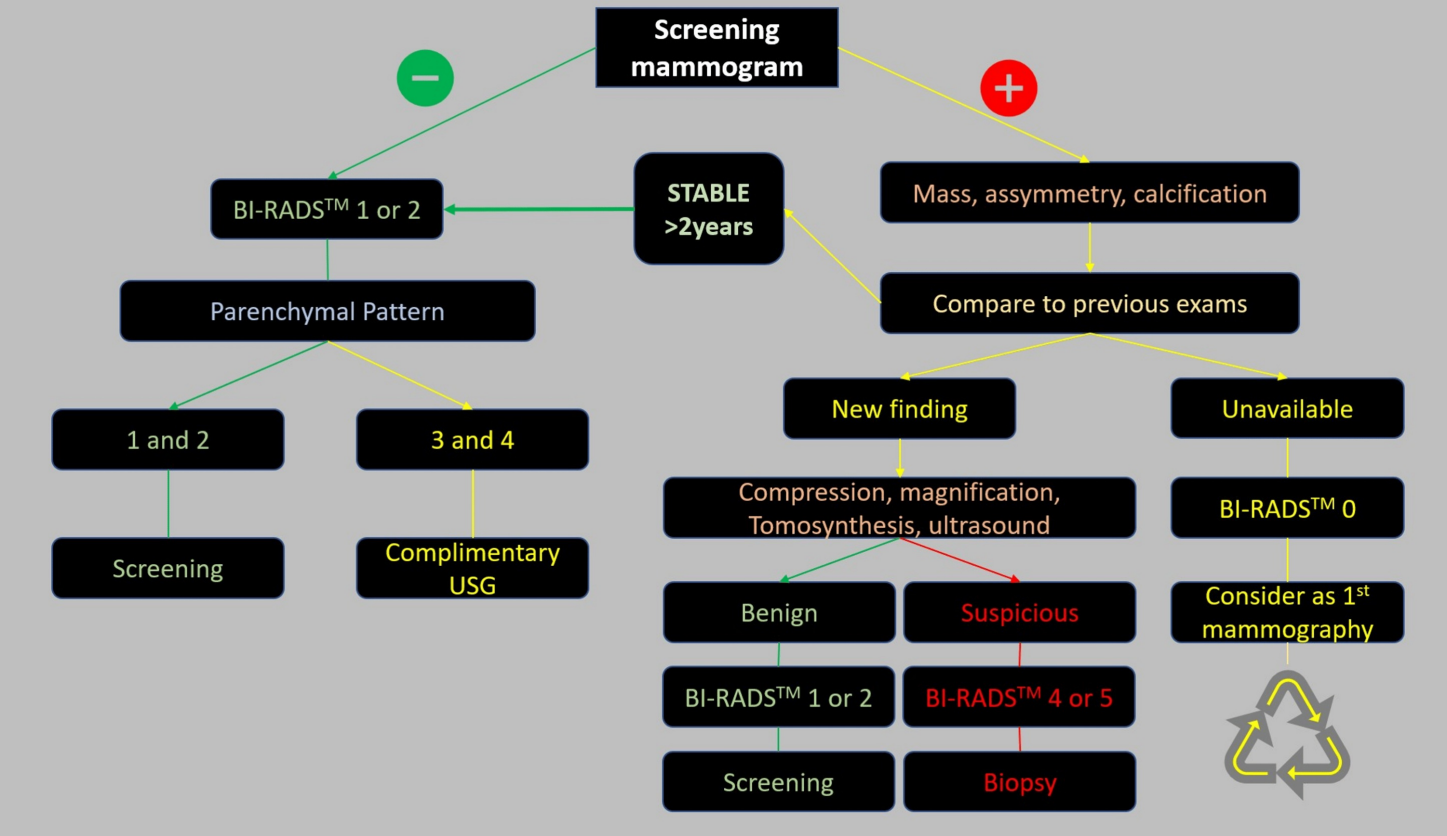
Workflow for screening mammogram We should not have BI-RADS® 3 classification. BI-RADS® 0, 1, and 2 at first assessment. After comparison to previous studies, benign or malignant assessment should be reported. When the previous exams are unavailable, a vicious circle should be formed. BI-RADS®: Breast Imaging and Reporting Data System, USG: ultrasonography
